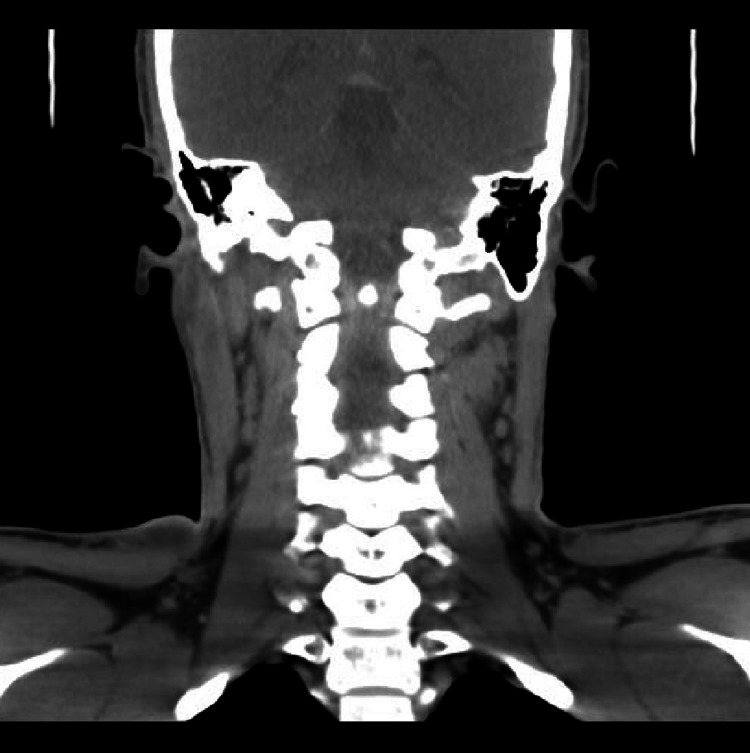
Coronal cut CT of the Neck showing multiple enlarged cervical lymph nodes.
